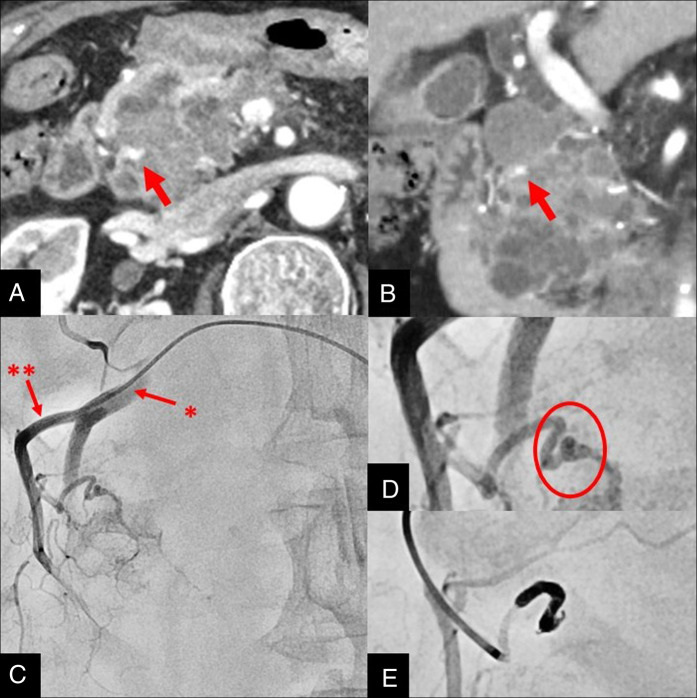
Contrast-enhanced computed tomography. (A) Axial and (B) coronal images demonstrating a pseudoaneurysm within the cyst (arrow). Abdominal angiography. (C) Overview, gastroduodenal artery* and posterior superior pancreaticoduodenal artery. (D) Magnified images confirming the presence of a pseudoaneurysm. (E) Coil embolization was performed.
